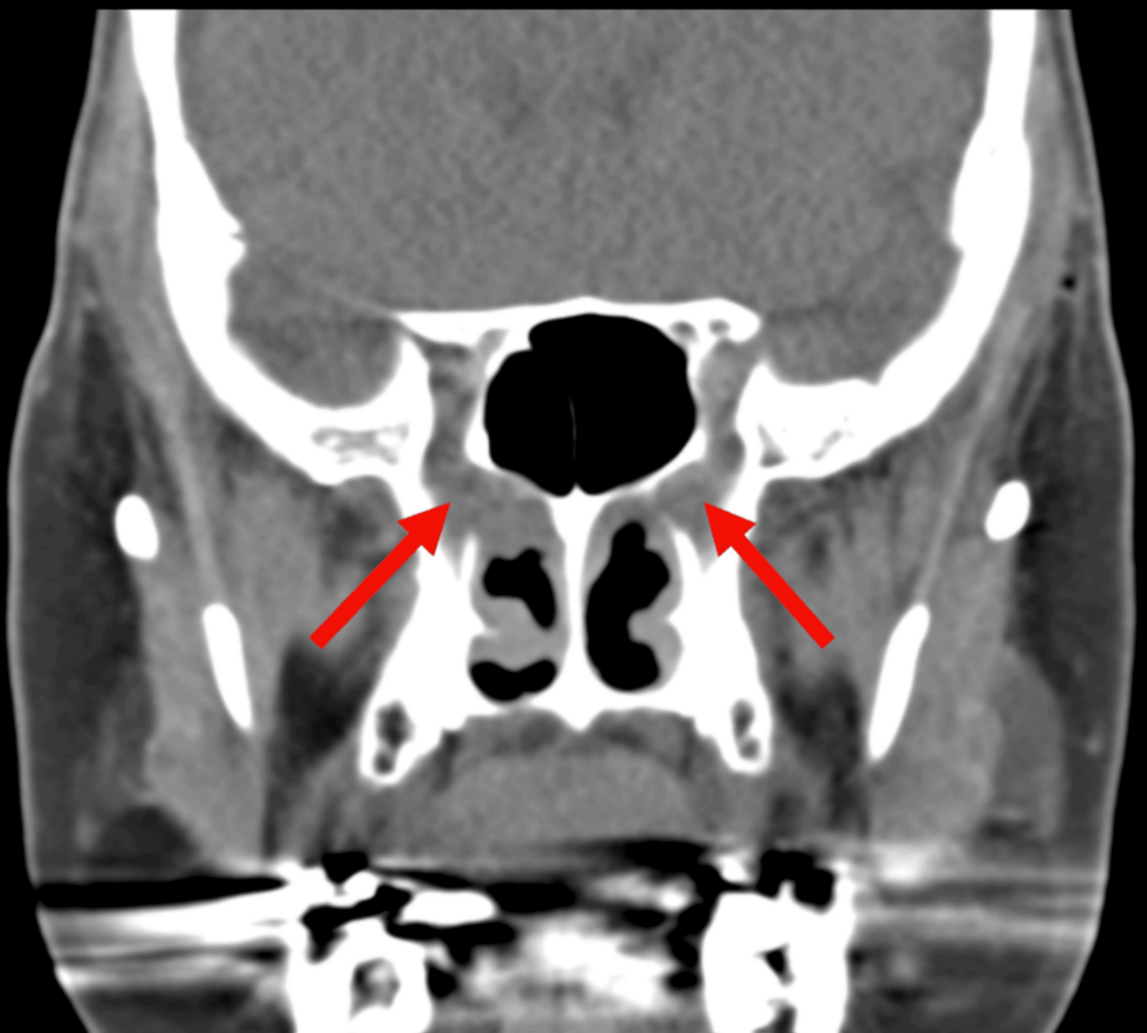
Coronal head CT with IV contrast demonstrating abnormal soft tissue thickening in the pterygopalatine fossa (arrows), extending into the sphenopalatine foramen, concerning for an infiltrative process. CT, Computed Tomography
